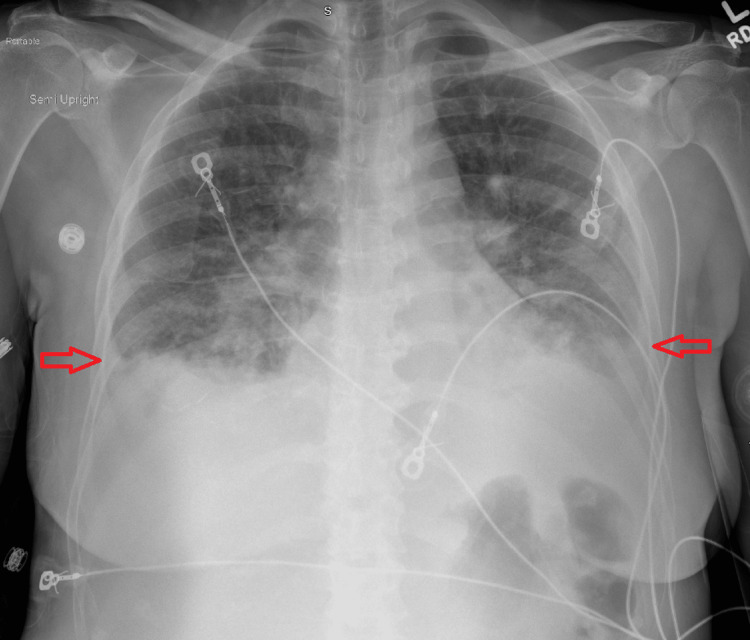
Chest X-ray anteroposterior view showing bilateral pleural effusions on the day of admission (shown by red arrows)
